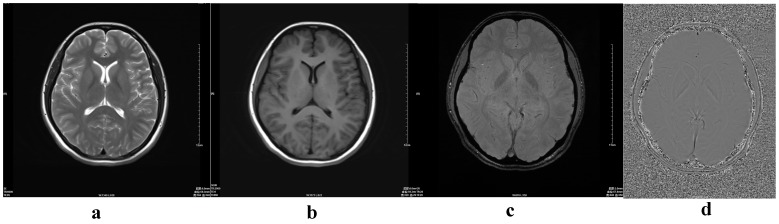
MRI findings in a 17-year-old normal girl. (a) axial T2-weighted image; (b) axial T1-weighted image; (c) magnitude image; (d) phase image.
